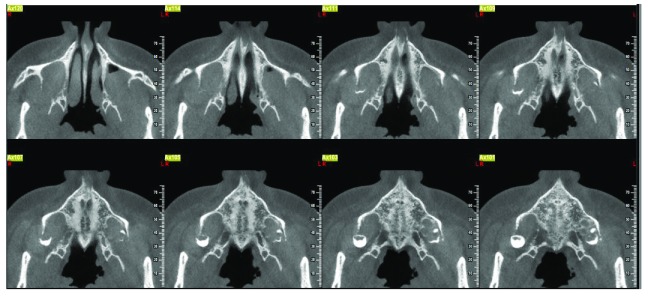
CT scan. Assial view showing solid mass in right maxilla causing destruction of the maxillary sinus floor.
